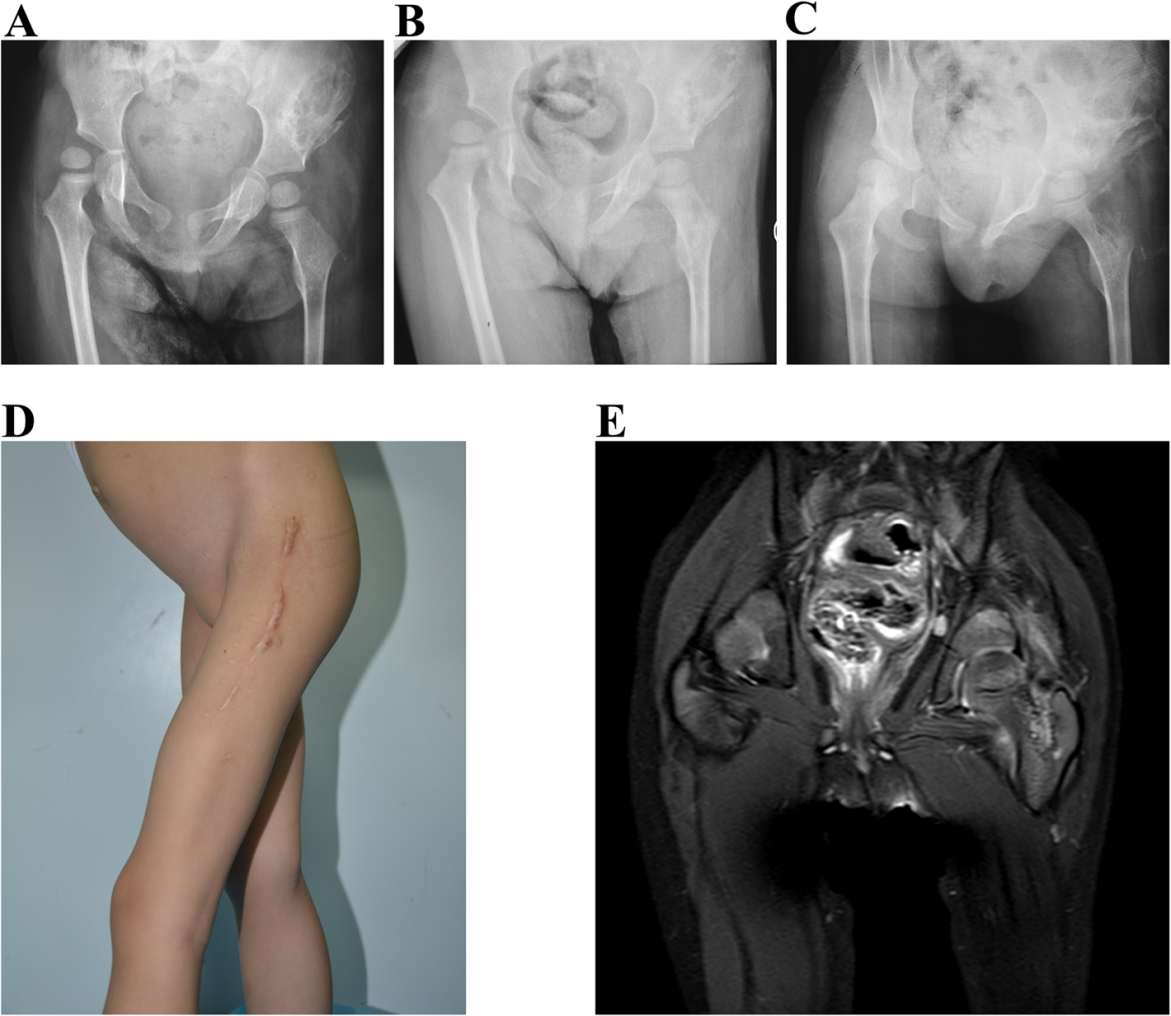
Deep KHE with bone and joint destruction in a 3.5-year-old girl. The patient had been diagnosed with a left-hip KHE associated with KMP at 1 year of age. She received a partial resection at the local hospital. Although surgical excision improved the associated KMP, she exhibited a progressively decreased hip range of motion. An anteroposterior pelvis radiograph showed right hip subluxation and progressive bone erosion in the left ilium and proximal femur before referral (a, b and c). Coronal T2-weighted MRI revealed a deep lesion infiltrating the left ilium and proximal femur at the time of referral to our department (d, e)
